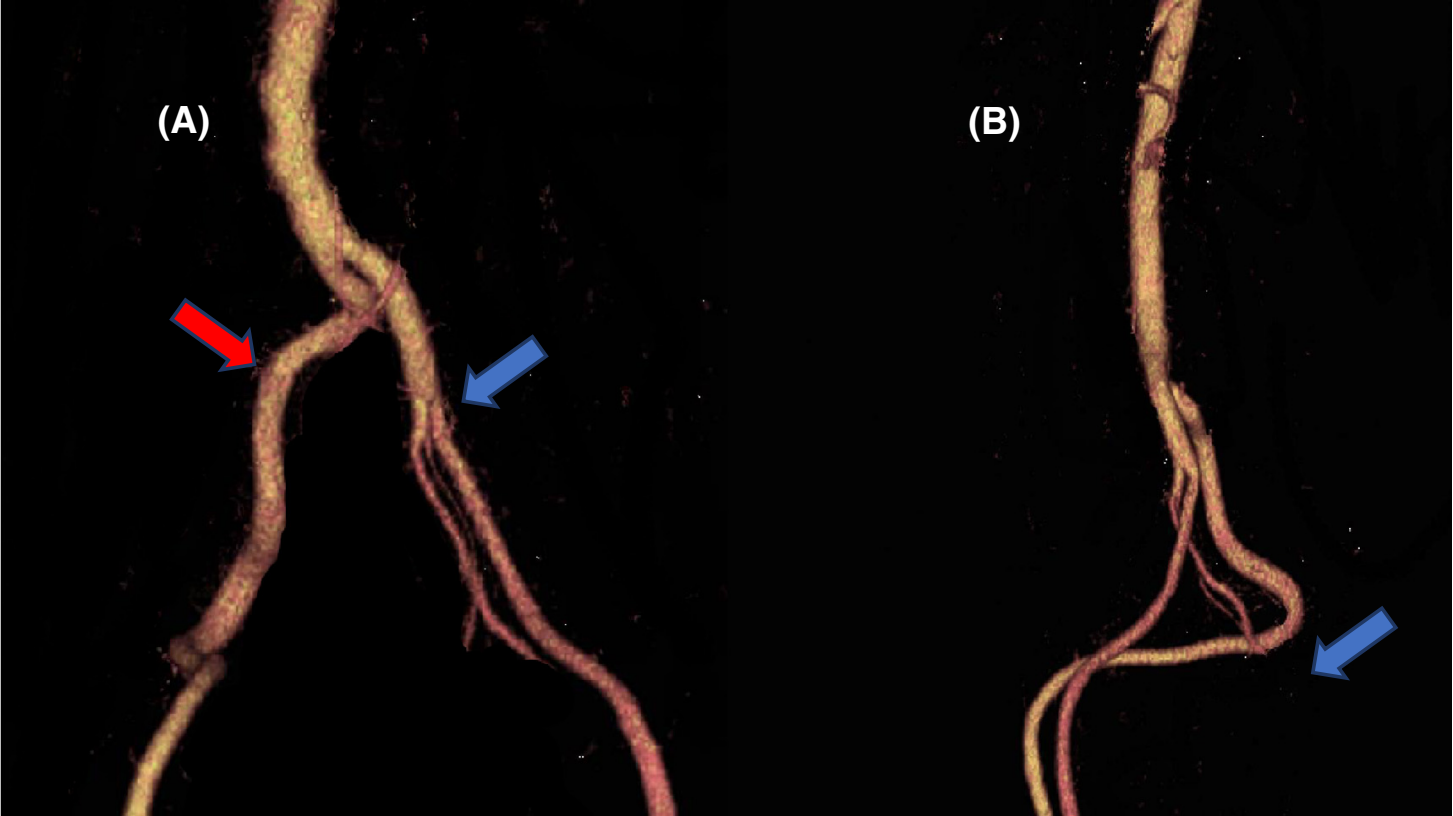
Three‐dimensional CT of aorta. (A) The abdominal aortic branch in front of the fourth lumbar vertebra. The left common iliac artery becomes a normal branch of the internal iliac artery and external iliac artery (blue arrow). However, the right common iliac artery is deficient in the external iliac artery (red arrow), as it flows directly to the internal iliac artery and descends along the front of the sacrum. (B) Lateral view of aorta. The angle of the abnormal blood vessel on the right is 34° (blue arrow).
